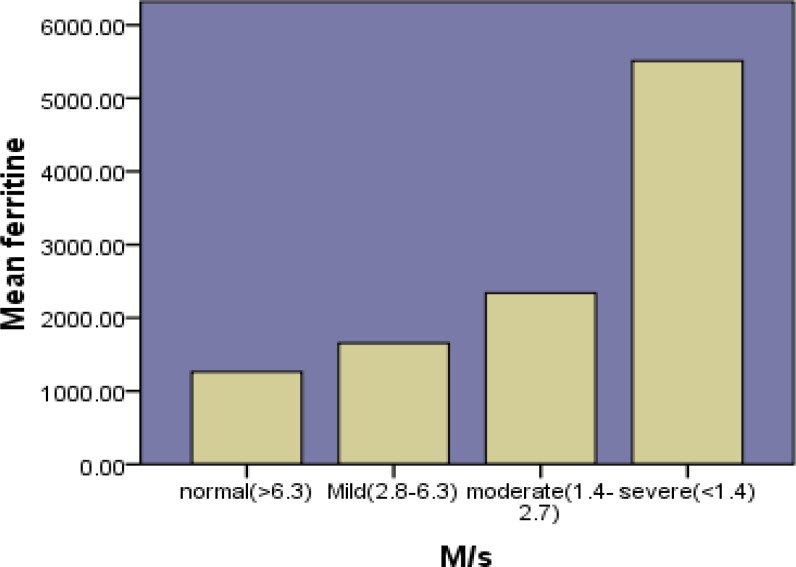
Serum levels of ferritin and hepatic T2*MRI
